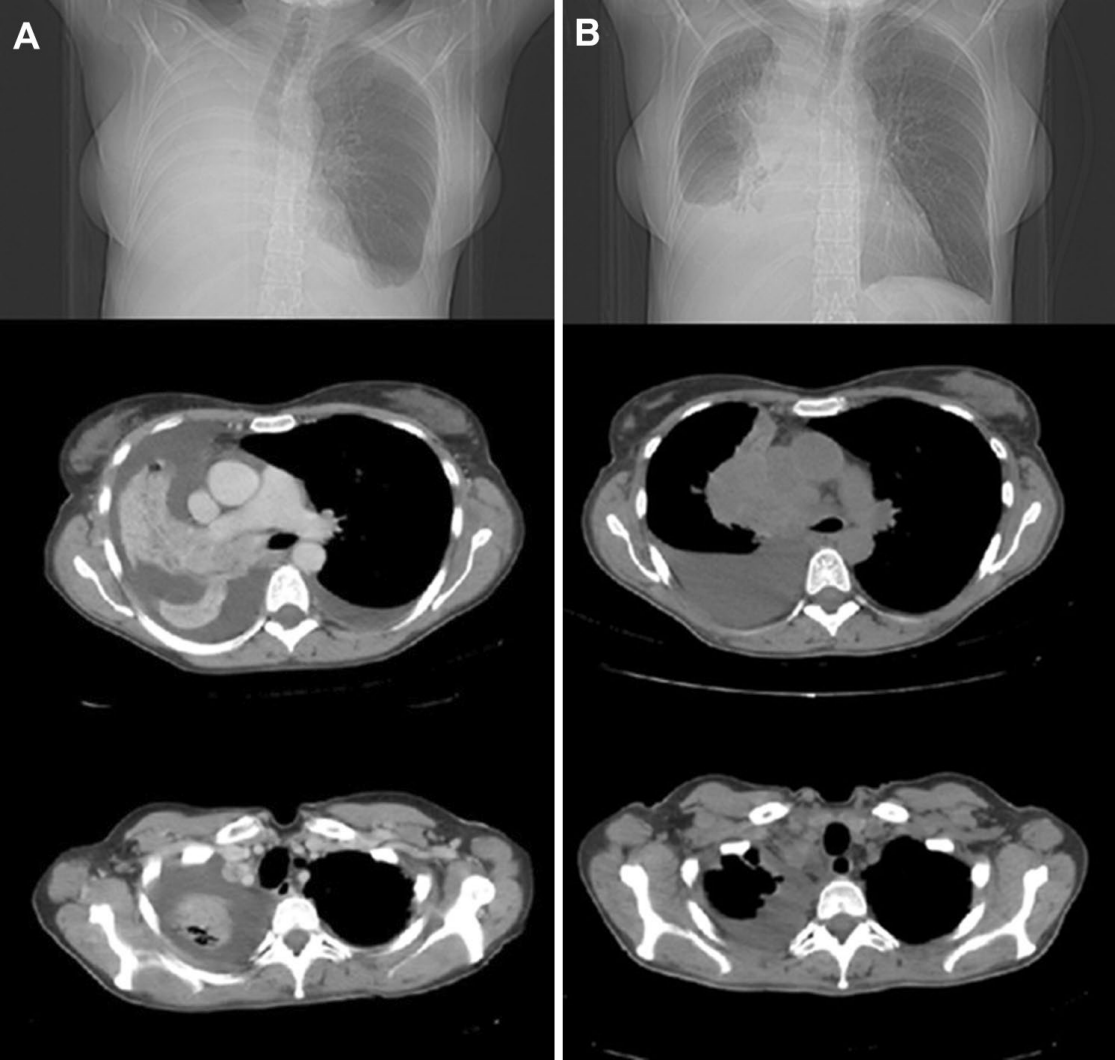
Chest X-ray and CT scan of a patient with locally advanced non-small cell lung tumor before (A) and after (B) accelerated-hypofractionated radiotherapy (20 Gy in four fractions). The patient reported complete response of dyspnea three days after the treatment.
